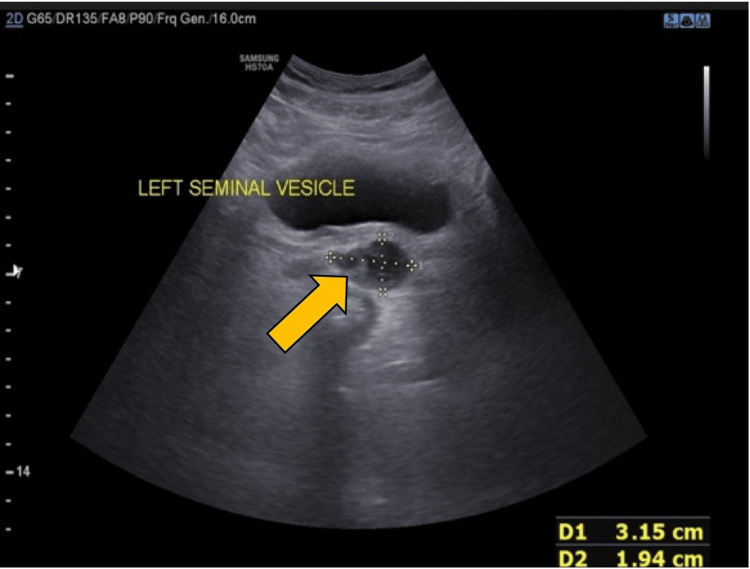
Ultrasonography showing a cystic lesion with multiple echoes in the left seminal vesicle, suggestive of seminal vesicle abscess
